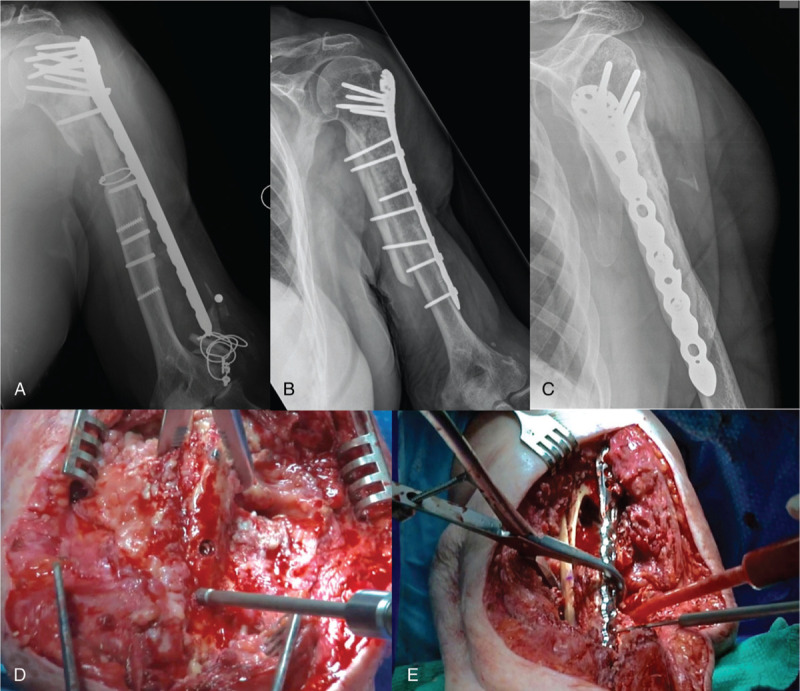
Proximal humeral nonunion and broken/failing hardware. Radiographs of an 80-year-old woman with ongoing pain and dysfunction of her left arm following multiple previous surgeries over the past year, demonstrating a proximal humeral nonunion and broken/failing hardware (A). She had no clinical signs of infection. Her infectious bloodwork demonstrated mildly elevated WBC, ESR, and CRP. She underwent revision surgery consisting of complete hardware removal, irrigation, and debridement with deep cultures and revision open reduction internal fixation of her left humerus. The broken screws were removed with a hollow reverse cutting reamer and conical extractor (D). A tibial strut allograft was used to provide additional stability across the nonunion and a proximal humerus locking plate was applied (E). In addition, cancellous morselized allograft and bone morphogenetic protein (BMP, Infuse, Medtronic) were applied at the nonunion/bone defect site. All of her intraoperative cultures were negative at 14 days. Follow-up x-rays at 1 year demonstrate bony union and the final construct (B and C).
